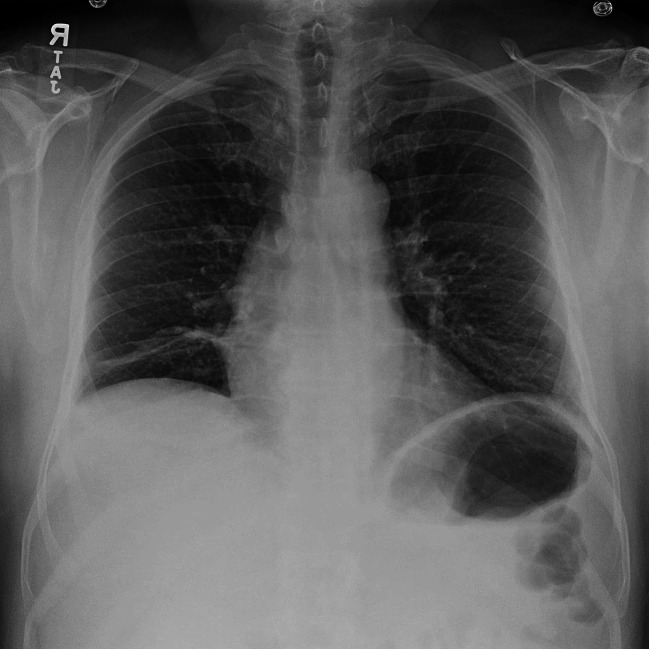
Chest radiograph showing elevated right hemidiaphragm
